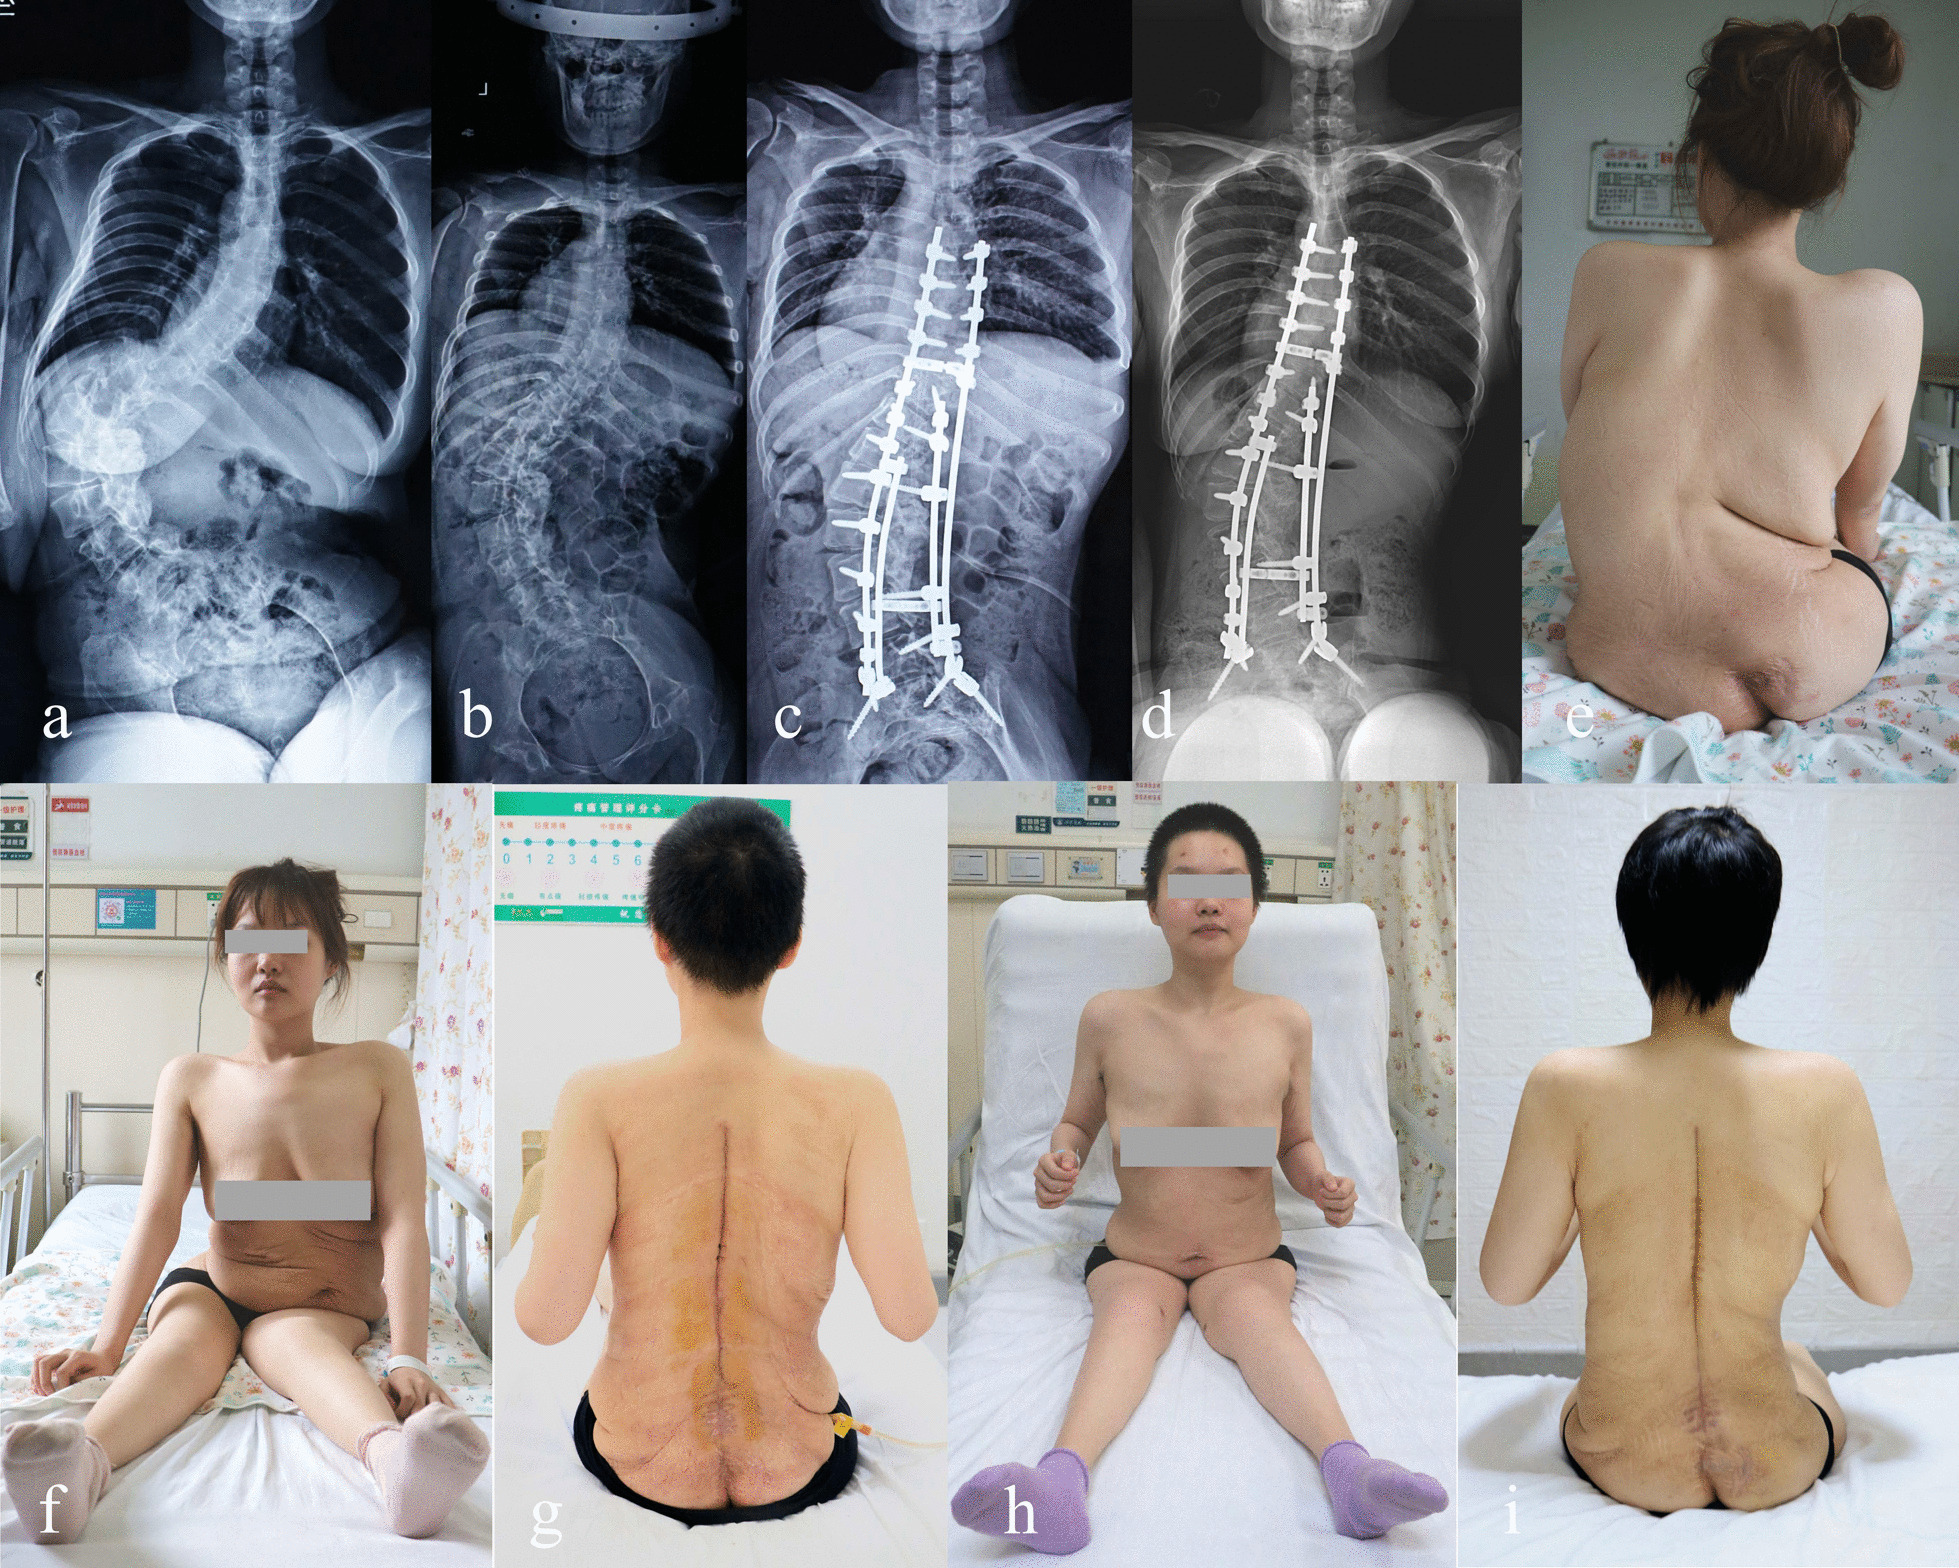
A 25-year-old female with SCI, NMS with severe PO. a Preoperative radiographs showed that the coronal Cobb angle of the major curve was 125.4° and PO was 43.2°. b After HFT, the coronal Cobb angle was reduced to 87.4° with a correction rate of 30.3%. c After MRPCO, postoperative radiographs showed that the coronal Cobb angle was further reduced to 53.8° with a correction rate of 57.1% and PO was reduced to 8.9° with a correction rate of 79.4%. d Postoperative radiographs at 66 months after surgery showed that the coronal Cobb angle was 56.4° with a correction rate of 55.02% and PO was 9.4° with a correction rate of 78.24%. e–i At the preoperative, postoperative and final follow-up stages, the patient’s trunk and sitting balance and cosmetic appearance showed remarkable improvement, which giving greater independence to use her upper limbs for daily activities
